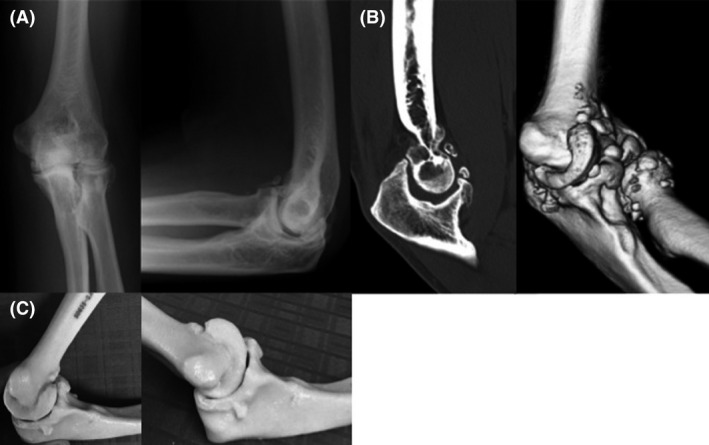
Radiography (A) and CT (B) showed the presence of bony protuberances. Estimated ROM was 130°of flexion and −20° of extension (C).
